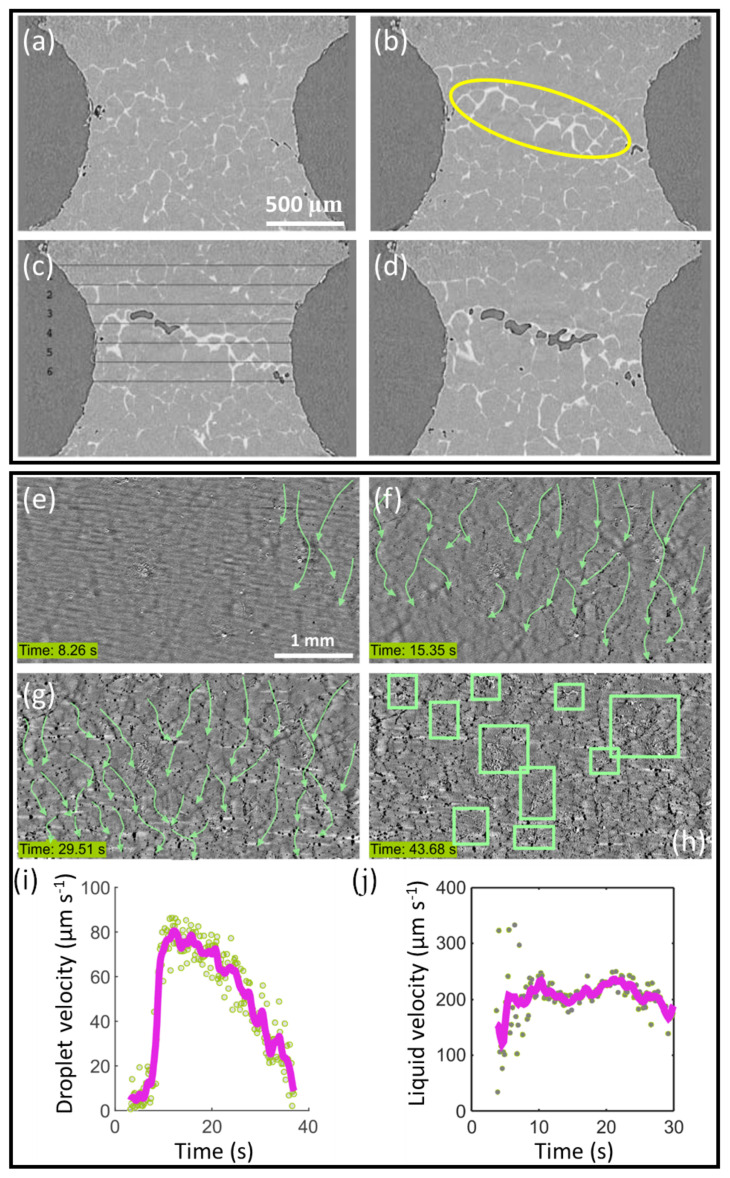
(a–d) Longitudinal section of a tomograph sequence showing the microstructure of semi-solid Al-8wt%Cu under uniaxial tension [128]. (e–g) A radiograph sequence of emulsified Pb droplets moving down the inter-dendritic liquid channels between pre-developed equiaxed α-Al dendrites, following paths indicated by the green arrows. Time t=0 s was set when an α-Al dendrite started to form in the field of view. (h) A radiograph showing hot tears (indicated by green boxes) formed towards the end of solidification. (i) The mean Pb droplet velocity as a function of time. (j) The estimated velocity of the liquid flow as a function of time.
